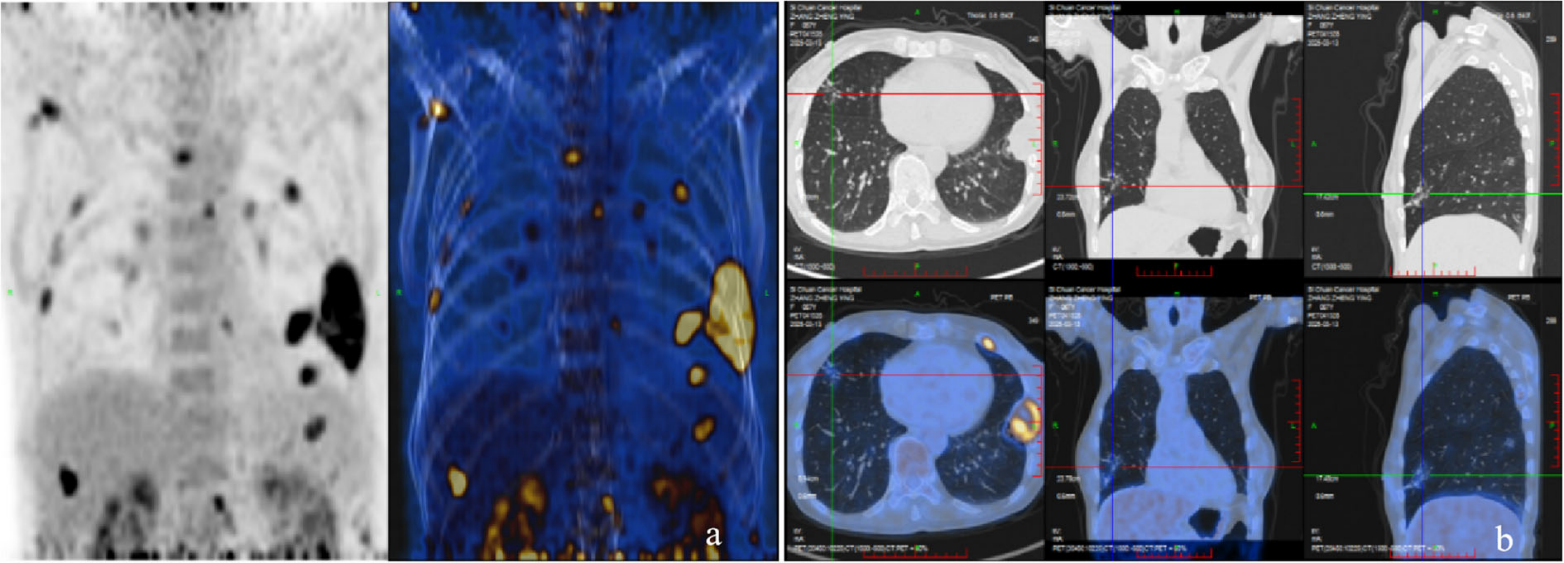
The overall condition (a) and condition of chest lesions (b) of the patient on PET CT.
